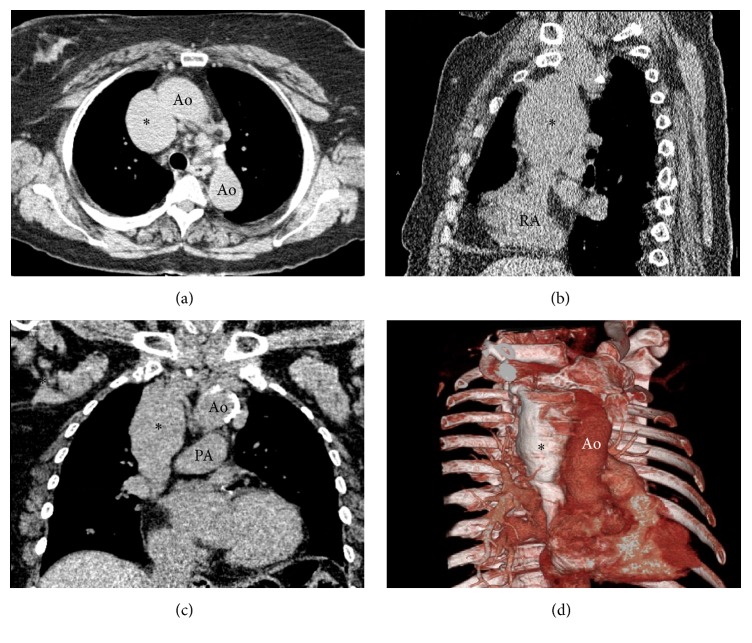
Axial (a), sagittal (b), and coronal (c) noncontrast CT images of the thorax demonstrating the SVC aneurysm (*∗*). (d) 3D reconstruction of the SVC aneurysm using CT of the thorax with IV contrast performed in 2014. Ao: aorta; PA: pulmonary artery; RA: right atrium.
